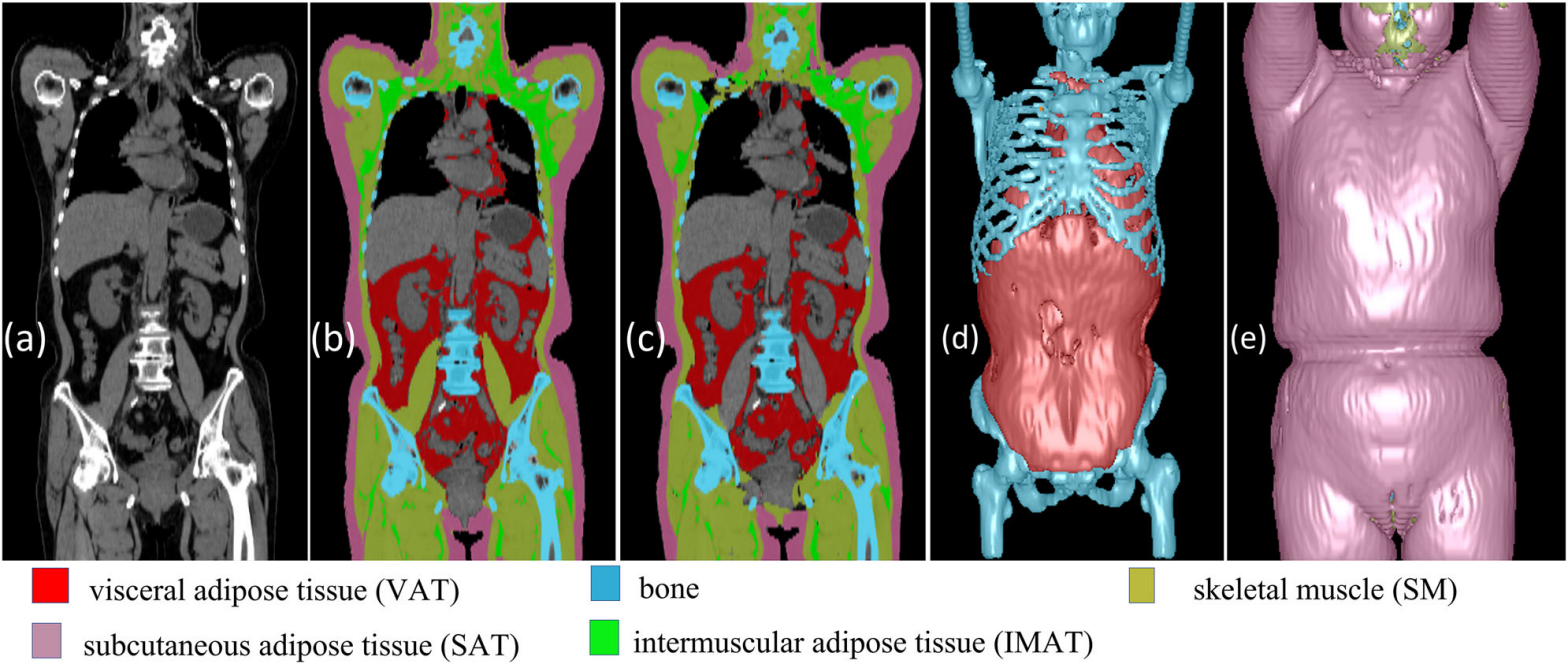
Computed results of body tissues on a whole‐body positron emission tomography‐computed tomography (PET‐CT) scan: (a) the original CT image, (b) the manual annotations of the body tissues, and (c) the computerized/automated segmentations of the body tissues. (d) and (e) The three‐dimensional (3‐D) visualization of the five body tissues
